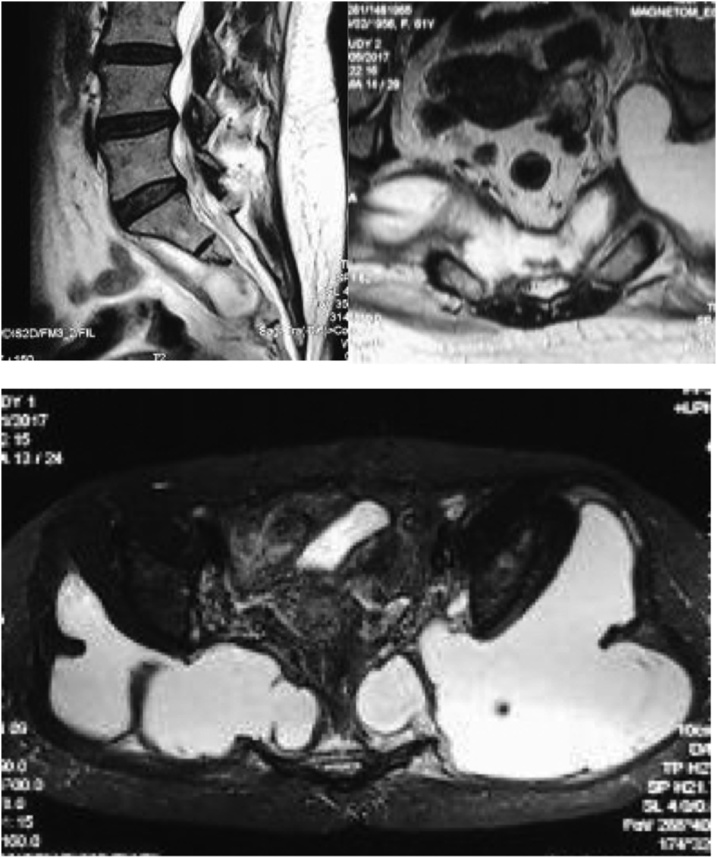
(a) T2-weighted sagittal MR images of sacral spine showing presacral abscess on distal sacral segment; (b) T2-weighted axial MR images on sacral spine (cut on the level of red line in image on the left) showing extension of the abscess to insertion of piriformis; (c) T2-fat suppressed axial images on pelvic showing further lateral extension on the abscess.
